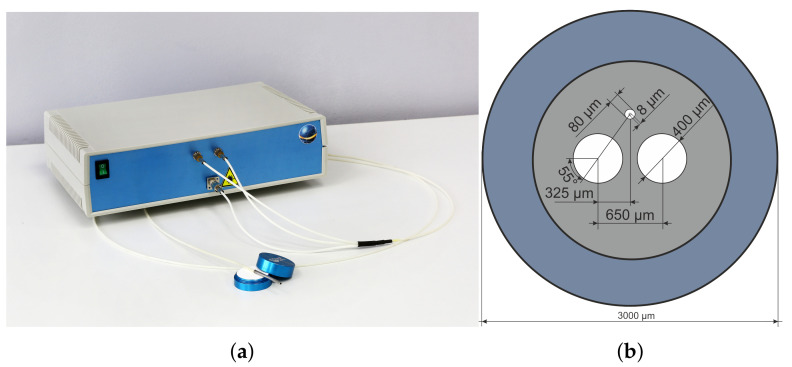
In-house built prototype of laser Doppler flowmeter with 2 mW of output light power, a signal amplifying, filtration unit, 50 kHz sample rate per channel (a) and fibre geometrical configuration (b).
